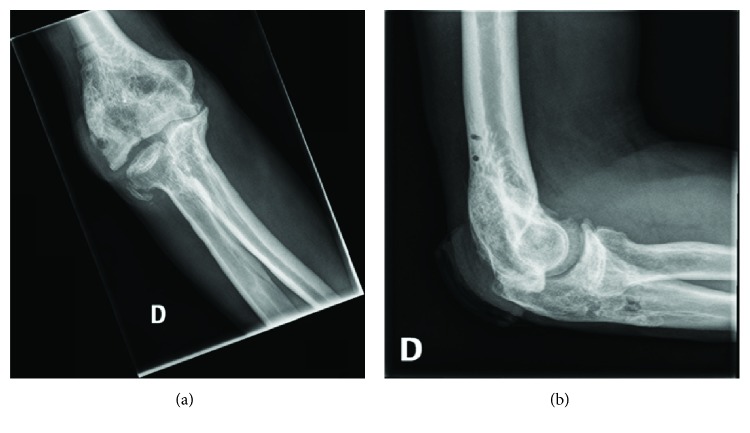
(a) Anteroposterior and (b) lateral 11 months postoperative X-rays of the right elbow.
